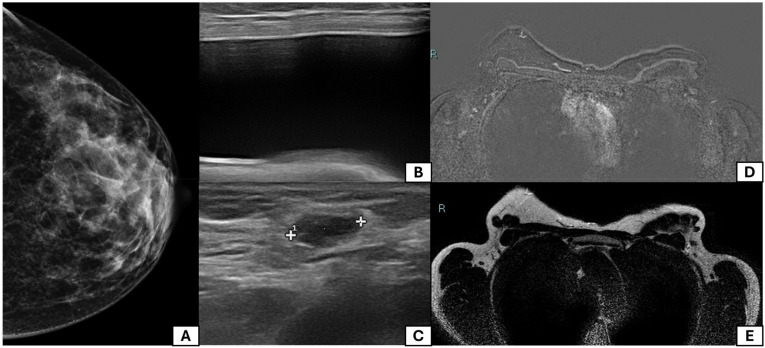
Lymph node recurrence at 54 months after nipple-sparing mastectomy in a 43-year-old patient with Grade 2 DCIS (Case 2). (A) Mammographic view showing pathological microcalcifications at diagnosis. (B) Post-operative ultrasound after nipple-sparing mastectomy with breast prosthesis placement. (C–E) Axillary ultrasound and MRI demonstrating the pathological lymph node in the axillary cavity; “+” markers and the number “1” represent ultrasound measurement markers for lesion size assessment.
